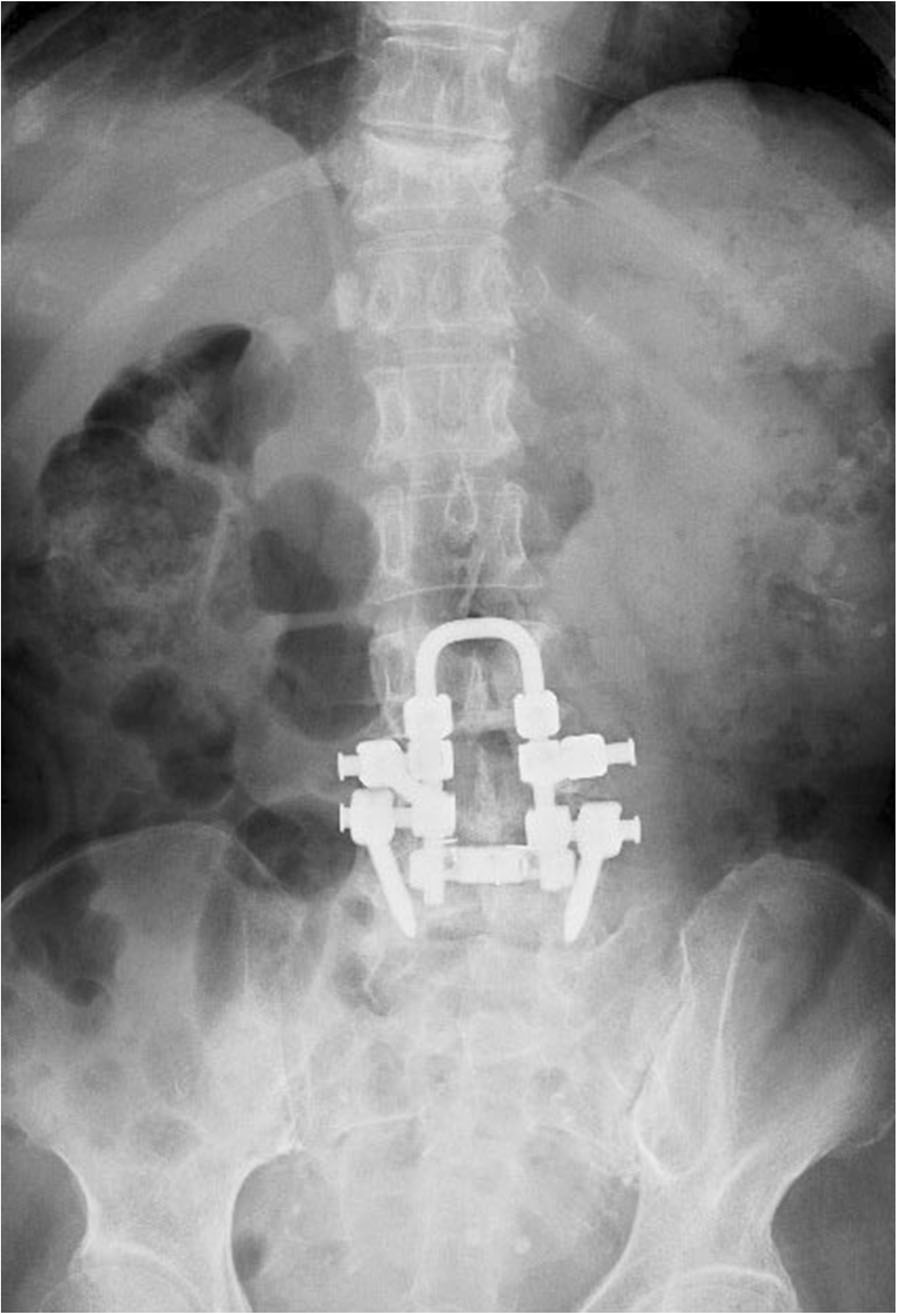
Radiograph showing lumbar spinal bone after two back surgeries in an elderly woman with failed back surgery syndrome
